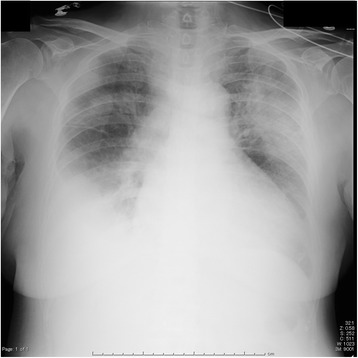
Chest radiograph indicated bilateral pleural effusion and pulmonary congestion
